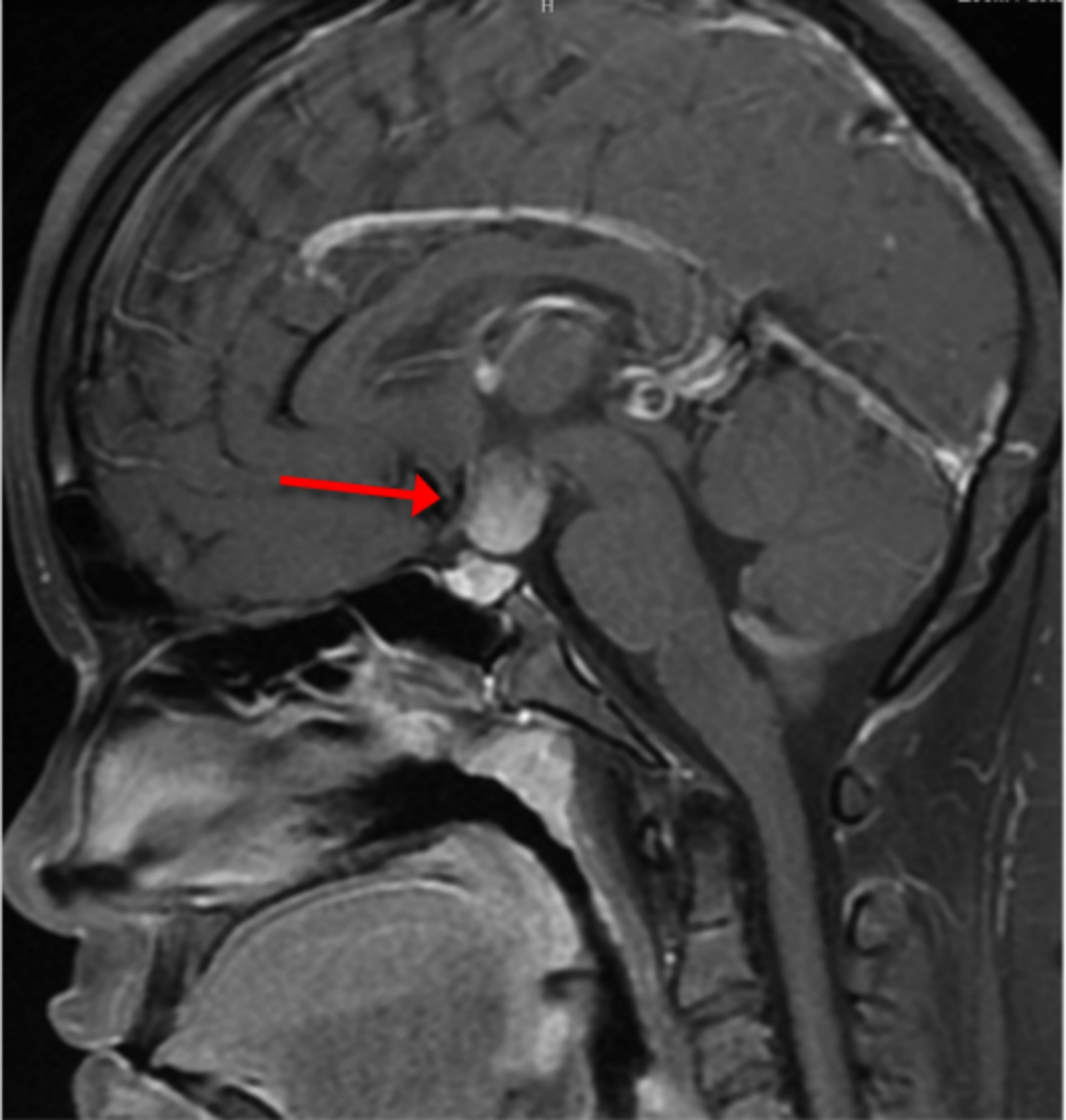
Pre-diagnosis MRI Scan Sagittal T1 post-contrast MRI prior to diagnosis indicates presence of 1.6 x 1.2 x 1.2 cm suprasellar mass.
